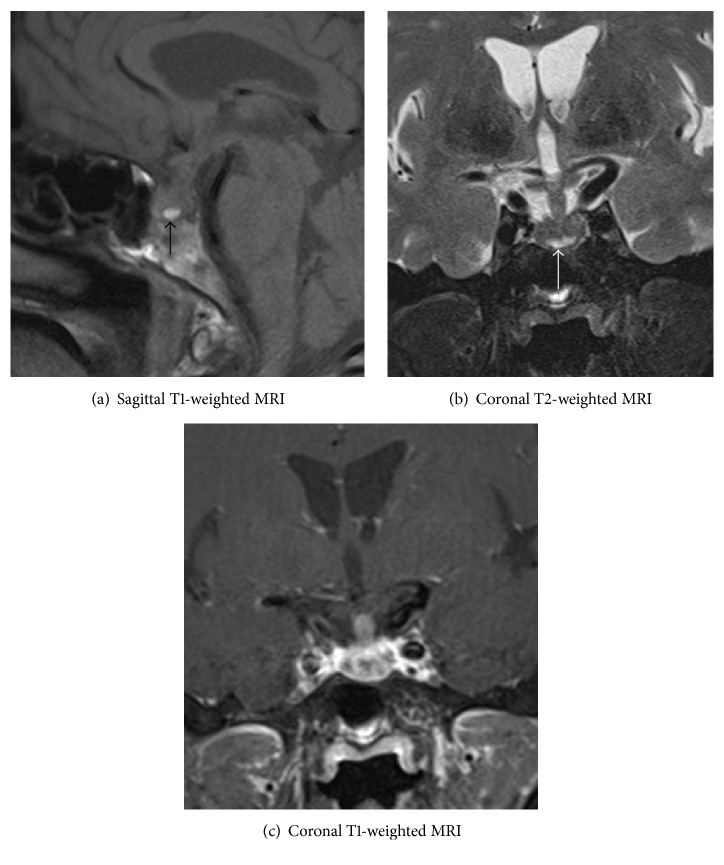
Inhomogeneity of the pituitary indicative of local metastases. Sagittal T1-weighted MRI (a) and coronal T2-weighted MRI (b) of the pituitary showed signal increase suggesting hemorrhage (arrows). Coronal T1-weighted MRI (c) with contrast shows inhomogeneous enhancement of pituitary with metastasis.
